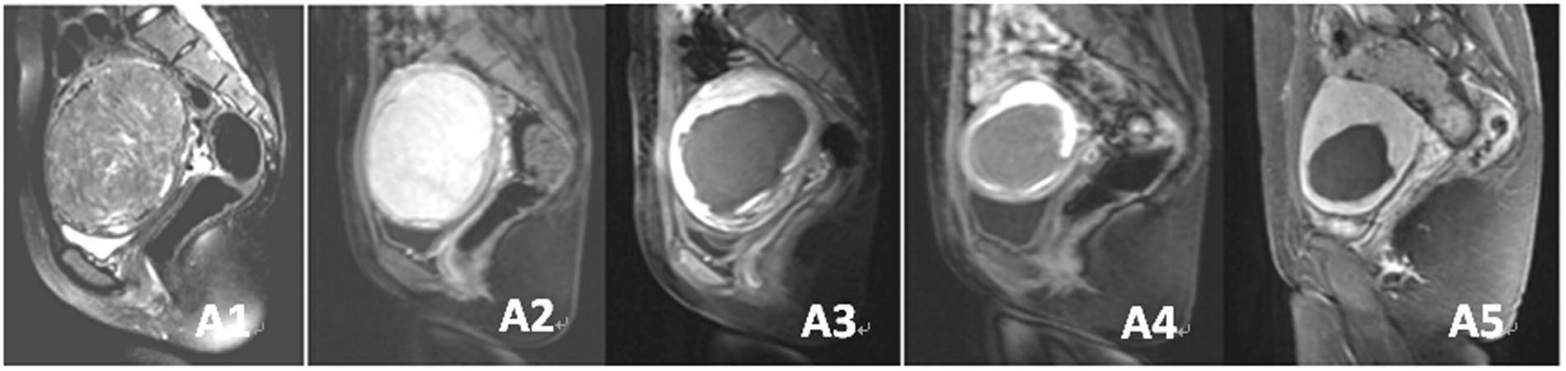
MRI of hyperintense fibroid before and after HIFU treatment: A1, T2WI-MRI before treatment; A2, fibroid shows enhancement on contrast-enhanced MRI before treatment; A3, 3 months after treatment; A4, 6 months after treatment; A5, 12 months after treatment.
